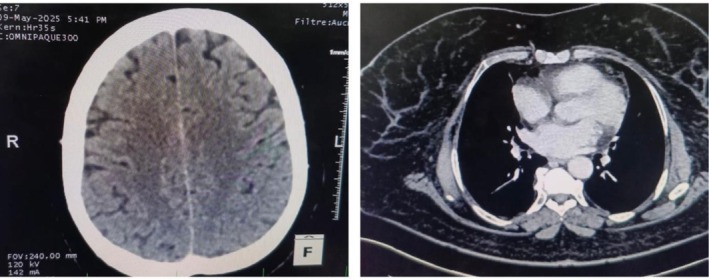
Representative thoracic and brain CT images demonstrating the absence of pulmonary cysts and intracranial lesions in this patient.
